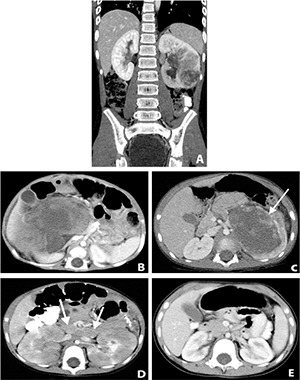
A, Ten-year old African-American male with sickle-cell trait. Coronal CT scan shows a centrally located and infiltrating left renal mass. Nephrectomy was performed and surgical pathology came back as renal medullary carcinoma. B, Large right renal mass in a 7-month old male. Nephrectomy was performed and the surgical pathology revealed a rhabdoid tumor. C, Two -year old male with a large left renal mass indistinguishable from a Wilms tumor. The mass was a clear cell sarcoma on surgical pathology. D, Four-year old female with history of abdominal enlargement. The kidneys are enlarged and show multiple hypodensities. There are also enlarged retroperitoneal lymph nodes (arrow); E, Follow-up CT 5 months later after chemotherapy showing normal kidneys and resolution of the retoperitoneal lymphadenopathy.
